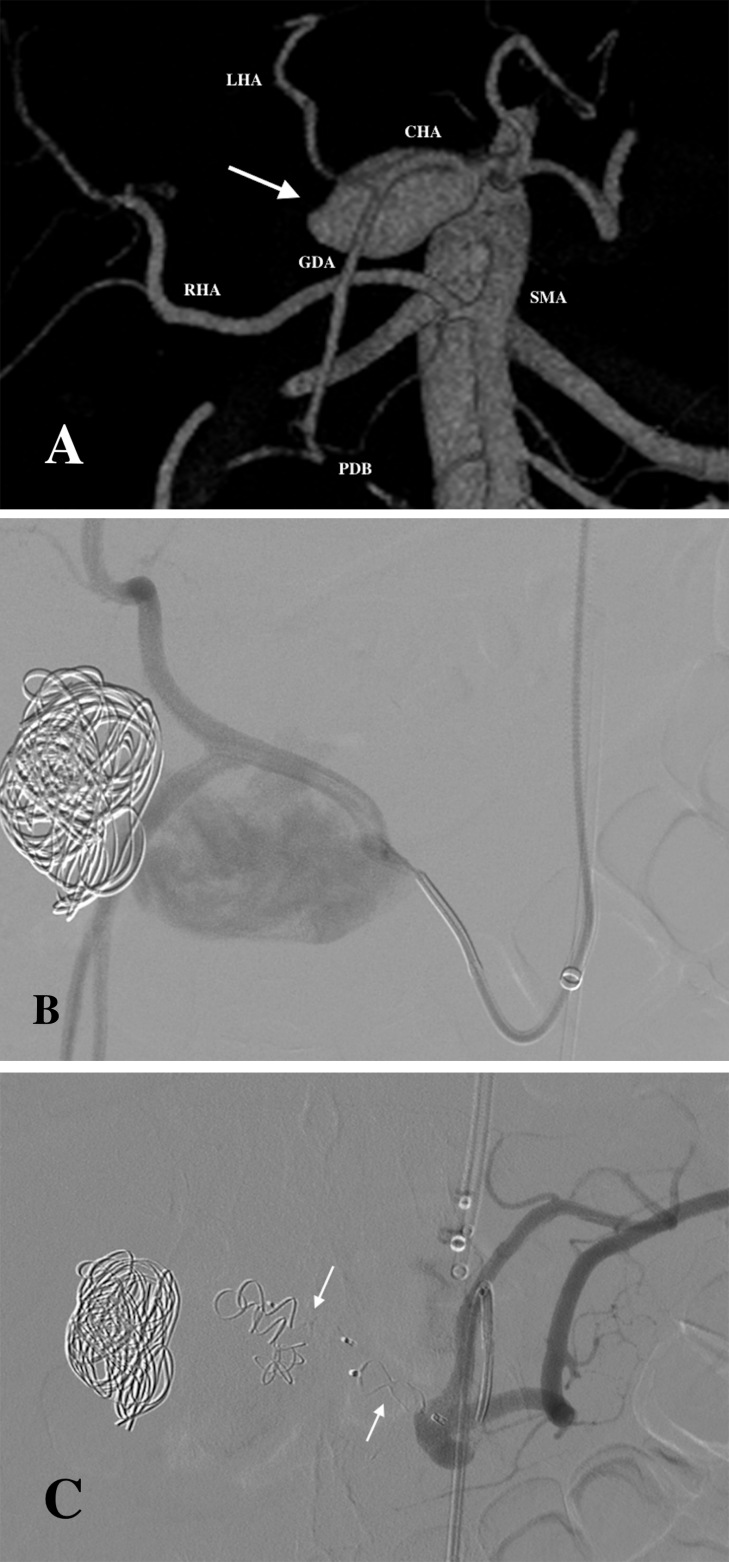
Pseudoaneurysm of the common hepatic artery variant treated initially with coil embolisation followed by vascular plugs placement. (A) 3D Volume Rendering CT image showing a pseudoaneurysm (arrow) arising from the common hepatic artery (CHA). This anatomical variant shows the right hepatic artery (RHA) arising from the superior mesenteric artery (SMA). The common hepatic artery branches into the gastroduodenal artery (GDA) and left hepatic artery (LHA). Patent pancreaticoduodenal branches (PDB) are present connecting the SMA and the GDA. (B) DSA image showing ineffective coil embolisation of the pseudoaneurysm. (C) Final DSA depicting procedural success after the placement of two vascular plugs.
